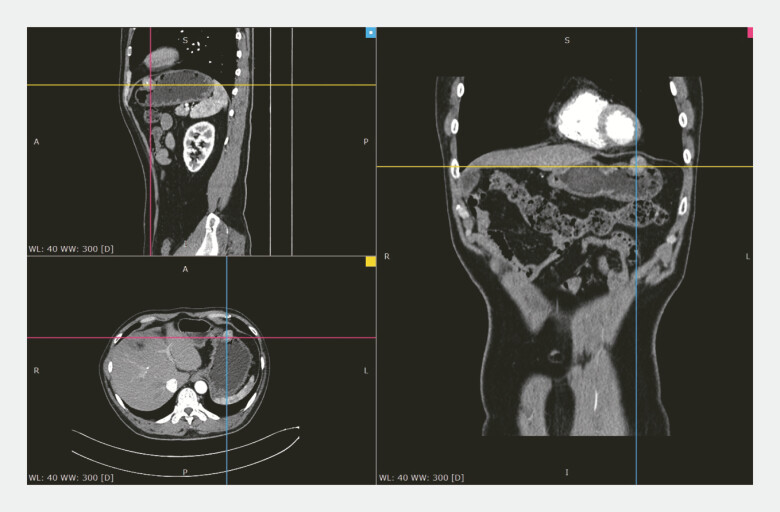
Abdominal enhanced CT showed the lesion arising from the gastric wall was projecting predominantly externally into the abdominal cavity. CT, computed tomography.
